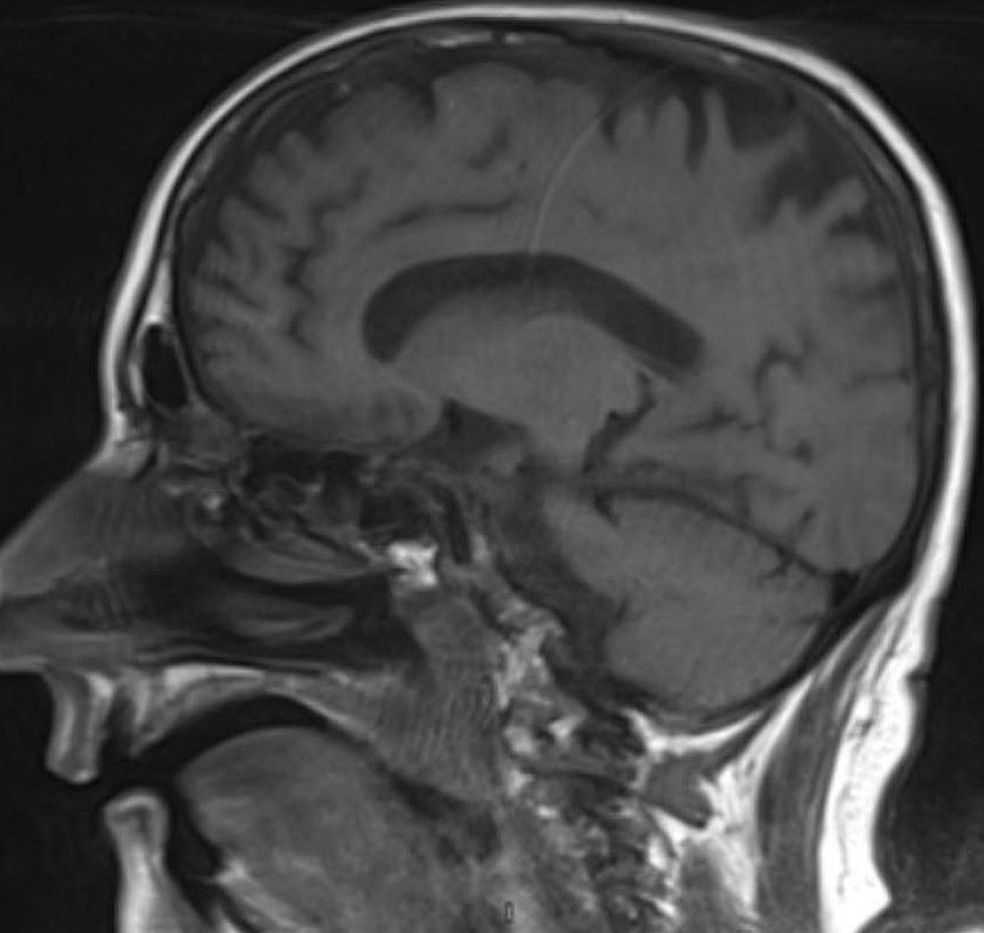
Sagittal T1-weighted magnetic resonance images of the brain showing low-intensity bone marrow signaling, consistent with bone marrow replacement or bone marrow proliferation disorder.
